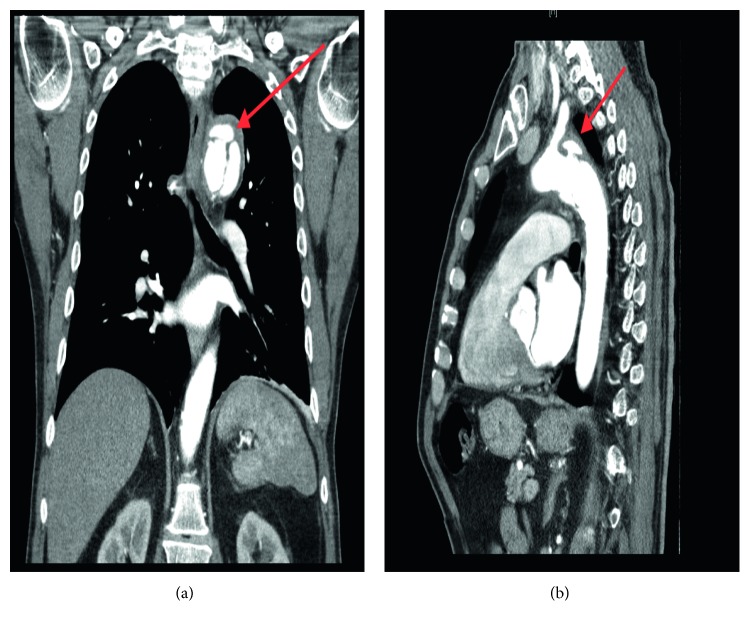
CT chest. Both (a) coronal and (b) sagittal views as indicated by red arrows showed type B aortic dissection with mycotic aneurysm.
